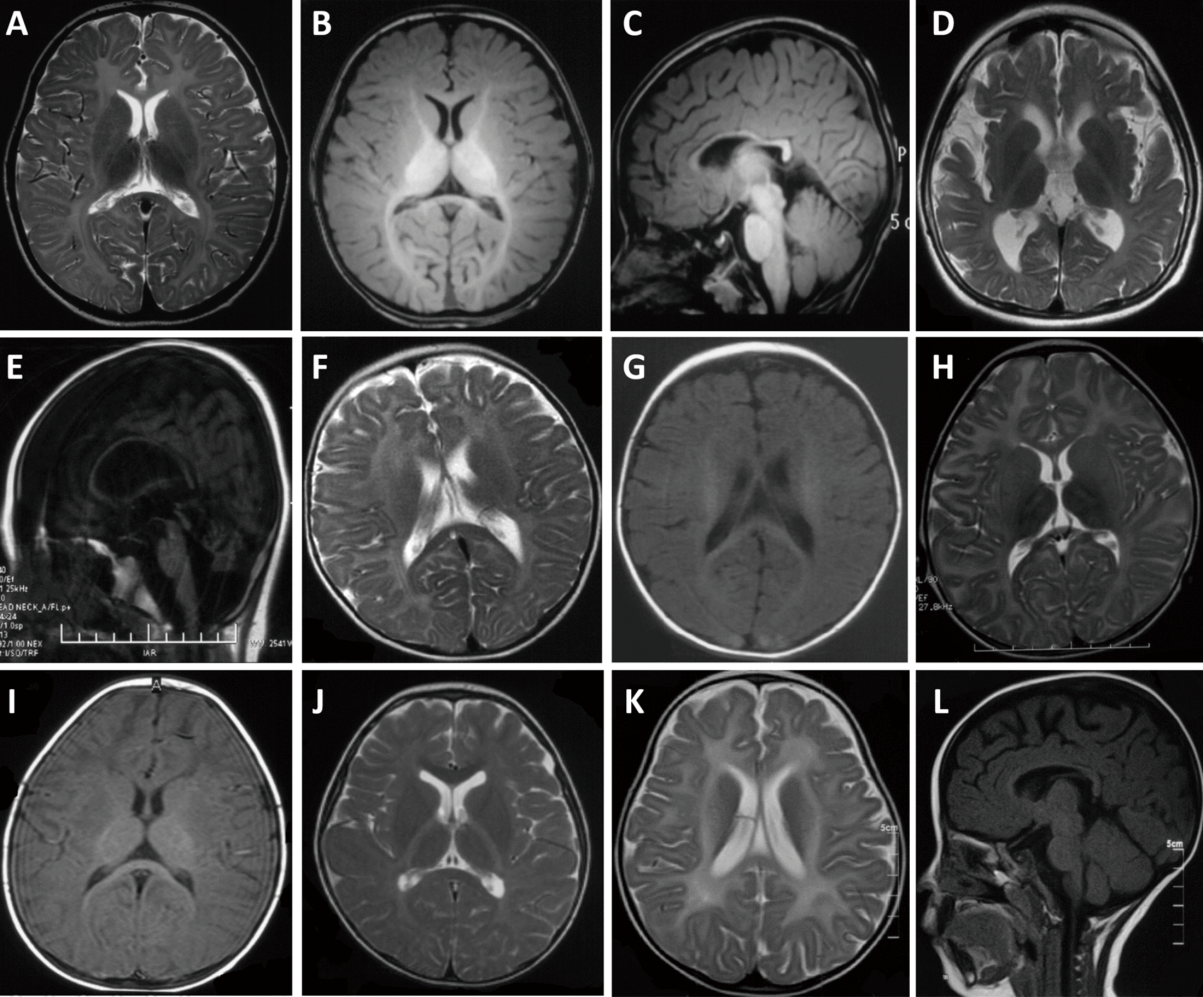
MRI findings of patients with hypomyelinating disorders. (A)–(C) P65 with PMD at 69 months of age. Homogeneous T2 hyperintensity in white matter and atrophy of corpus callosum is seen in axial T2WI (A) and sagittal T1WI (C). Axial T1WI (B) shows mild hyperintensity. (D)–(E) P106 with HABC at 7 years of age shows atrophy of cerebrum, relative preserved putamen, mild atrophy in caudate nucleus in axial T2WI (D), atrophy of cerebellum and corpus callosum in saggital T1WI (E). (F)–(G) P111 with GM1 gangliosidosis at 69 months of age showing T2 hyperintensity and cerebral atrophy. (H)–(I) P115 with GM2 gangliosidosis at 14 months old. T2WI shows diffuse hyperintensity in white matter and hypointensity in bilateral thalami (H), and mild hypointensity in white matter in T1WI (I). (J) Axial T2WI in P117 with Pol III-related leukodystrophy at 3 years of age showing diffuse T2 hyperintensity in deep white matter and posterior limb of internal capsules. T2 hypointensity was presented in anterior limb of internal capsules and corpus callosum. Axial T2WI (K) and saggital T1WI (L) of P118 with HLD9 at 18 months of age showing diffuse T2 hyperintensity in white matter and atrophy of cerebrum and corpus callosum.
